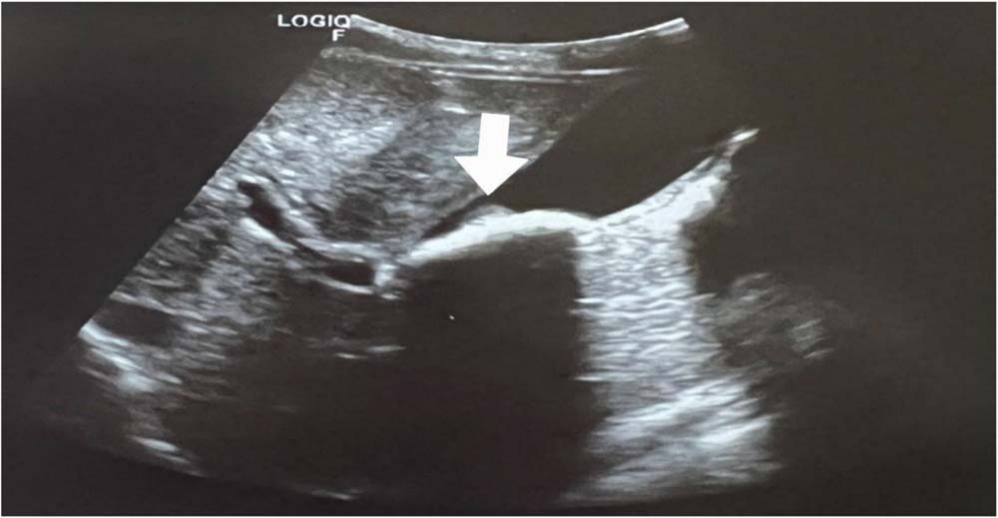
Axial view; ultrasonography of the gallbladder shows sludge with the stone formations (white arrow).
